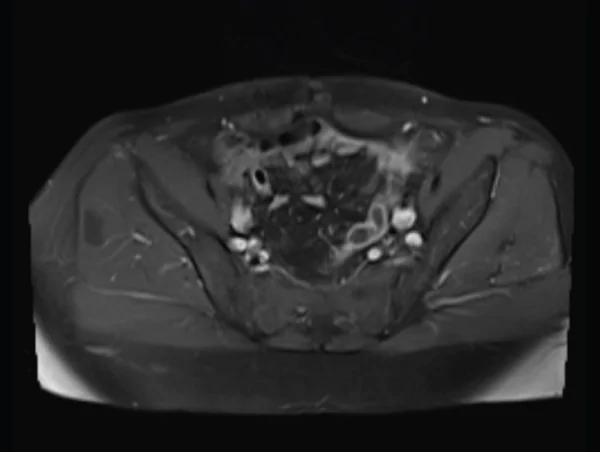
Posttreatment MRI with gadolinium, performed 5 years after treatment, showed no signs of recurrence.
